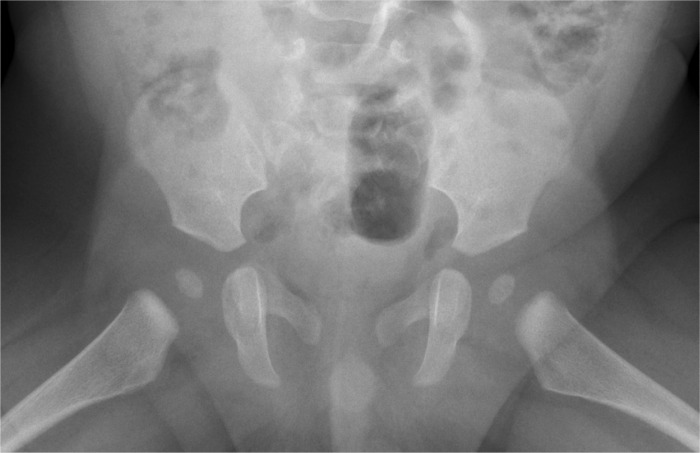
Representative FL radiograph demonstrating the acetabular sourcil as a single clear dense line, facilitating consistent landmark identification for AI measurement.
